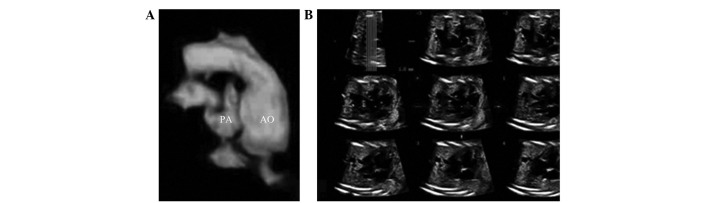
STIC image of the truncus arteriosus communis (Type I) of a 23-week fetus diagnosed by echocardiography. Reconstructed images of (A) the STIC reverse imaging mode and (B) the STIC TUI imaging mode. STIC, spatio-temporal image correlation; TUI, tomographic ultrasound imaging; AO, aorta; PA, pulmonary artery.
